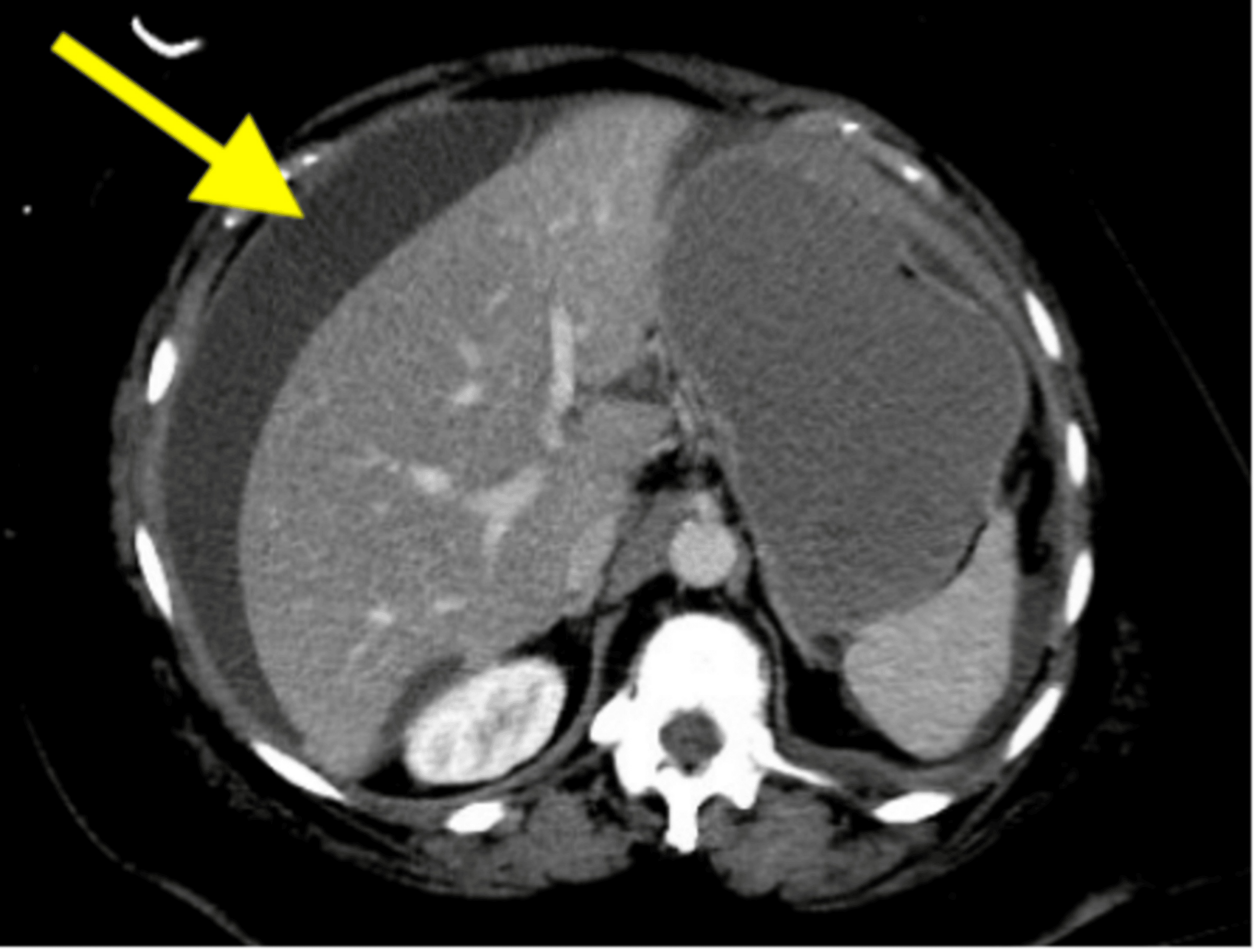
Axial cut of CT abdomen demonstrating a large volume of ascites with thickened and minimally nodular peritoneal enhancement, including thickening of the subdiaphragmatic left hemidiaphragm upwards to 13 mm.
